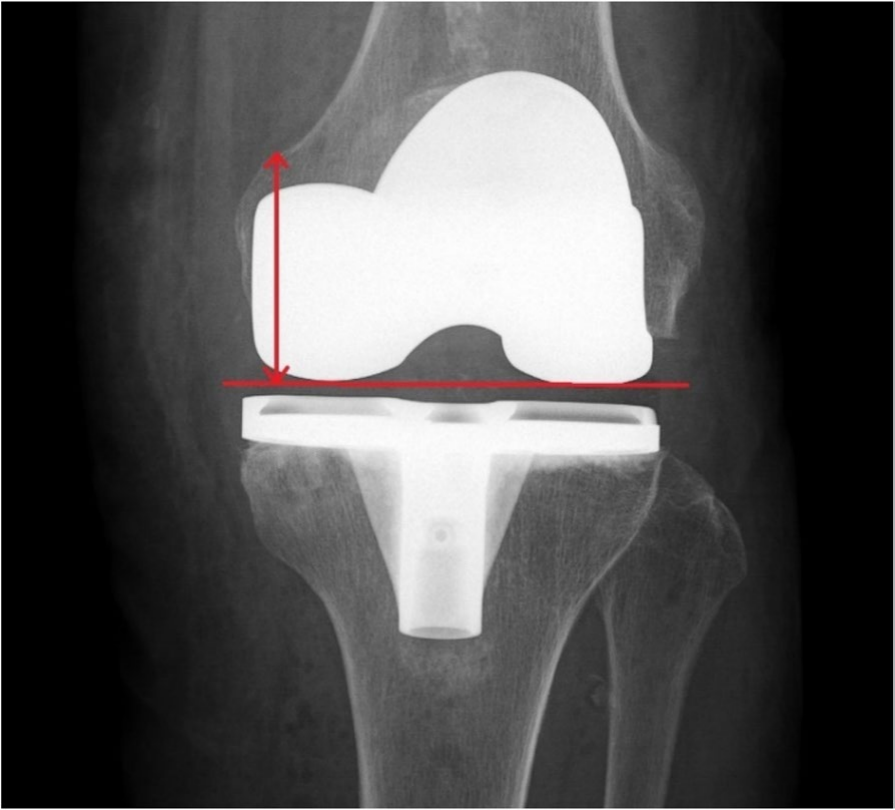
Measurement of postoperative joint line level on the weight-bearing antero-posterior x-ray
